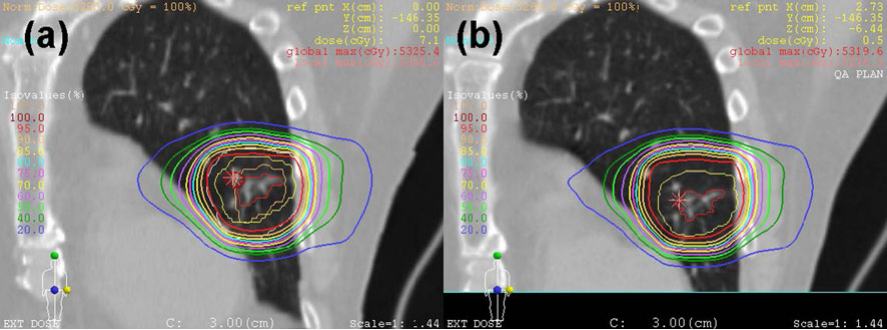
Examples of dose distributions for treatment planning (a) and 4D CT confirmation at gate-out (b). *Red*, *yellow*, *orange*, and *white* targets indicate GTV, CTV, IGTV, and PTV, respectively. The IGTV and PTV are contoured only on the gated CT images (a)
